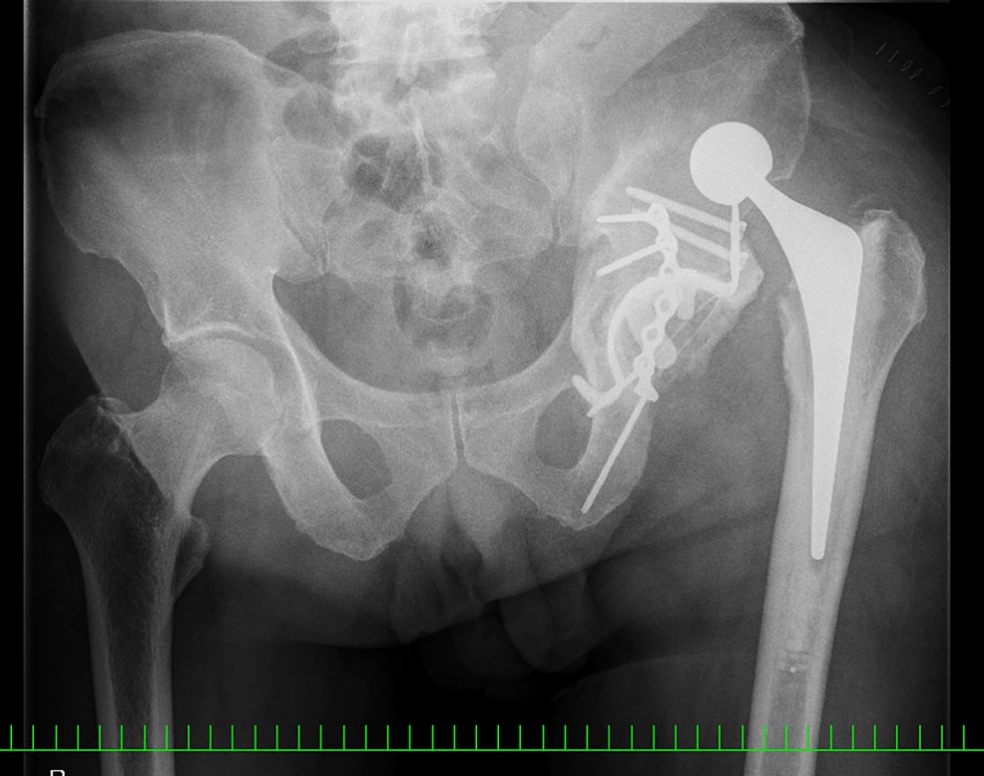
Plain radiograph one week after the first THA Posterior dislocation of the hip joint was revealed THA - total hip arthroplasty
